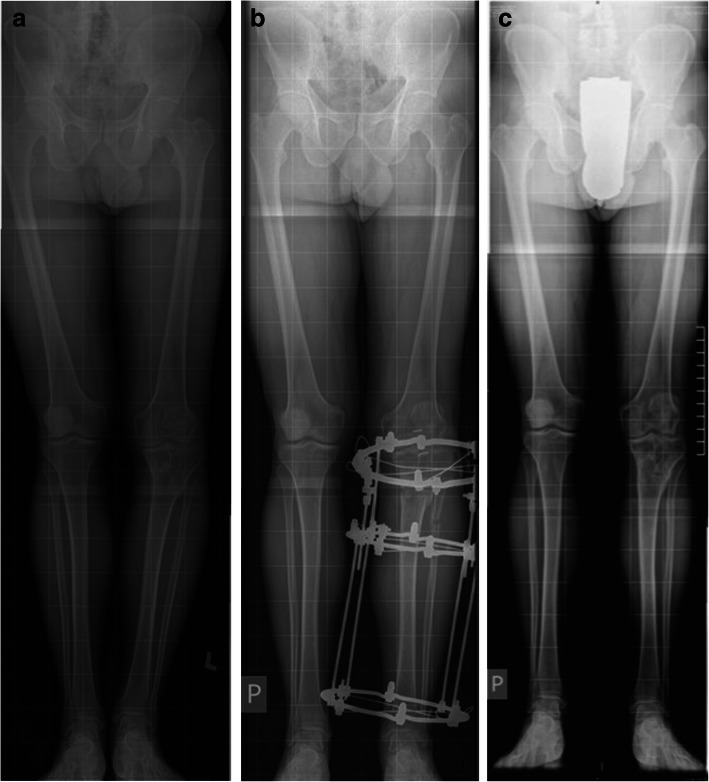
Computed radiography in a standing position. a Patient before treatment. b Patient during the treatment process. c Patient after treatment
